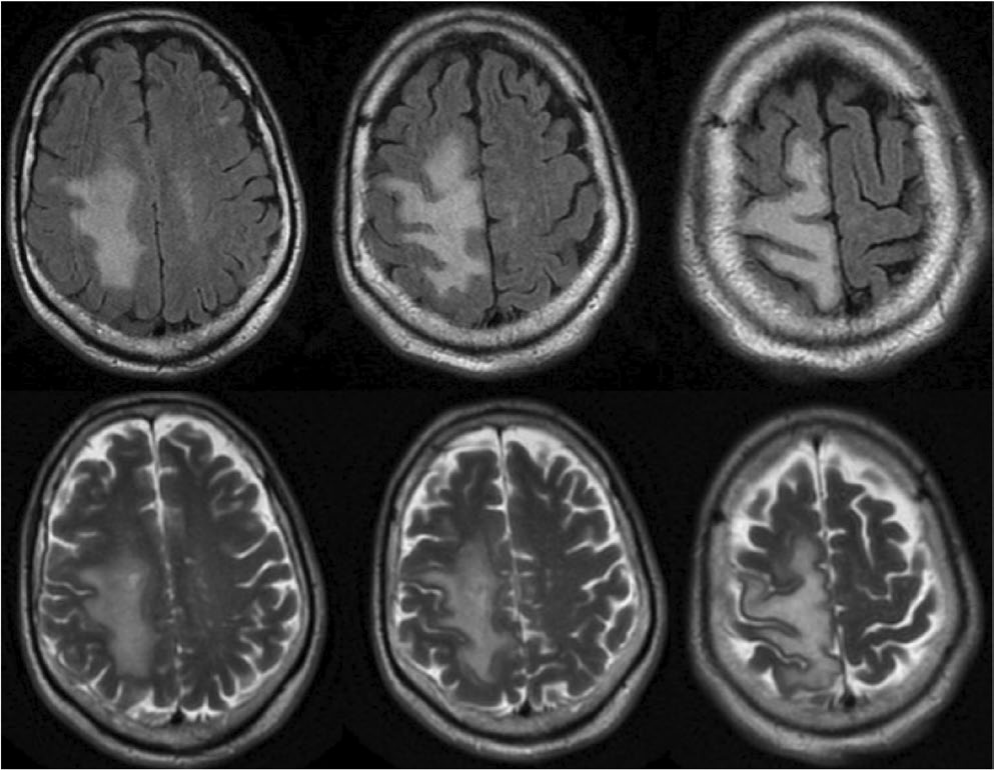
FIGURE 1 Axial FLAIR and T2‐weighted MRI before maraviroc treatment.
